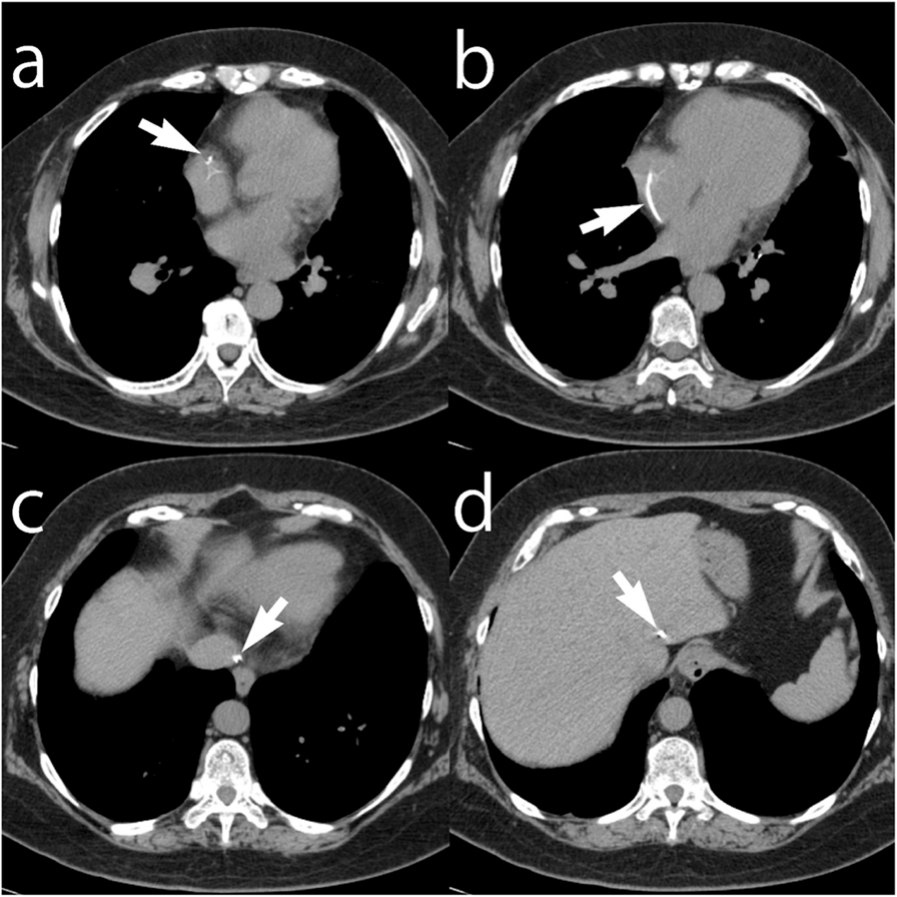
Non-contrast-enhanced CT images in a 61-year-old female patient with colon cancer show a dislodged central venous catheter fragment with inaccessible ends. The catheter was implanted 16 years ago to treat breast cancer and its fragment was migrated in the right atrium at least 3.5 years ago. a The upper end of the catheter fragment is located in the right atrial appendage. b It runs through the right atrium and c inferior vena cava until d its lower end strayed into the left hepatic vein
